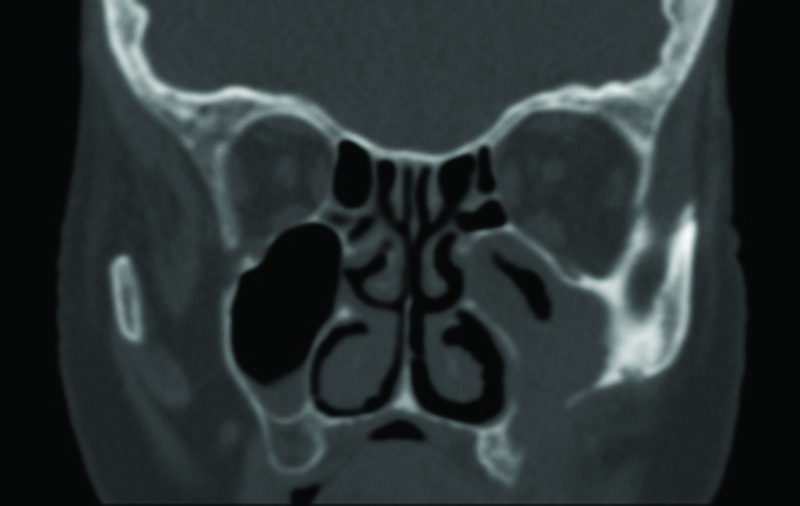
CT scan shows an opacification of left maxillary sinus with evidence of bone destruction of the anterolateral wall.
